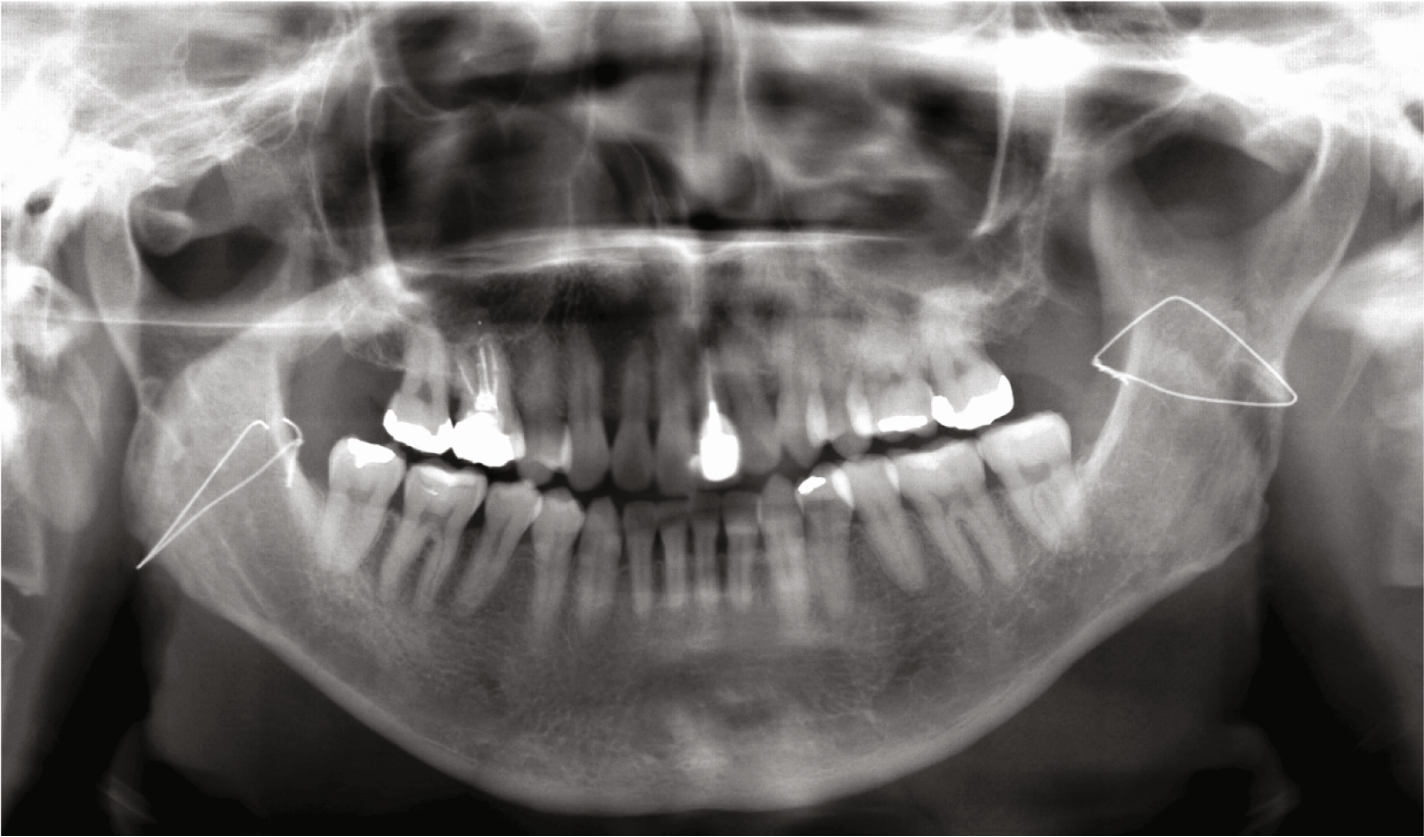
Panoramic radiograph shows normal findings except for the wires of prior orthognathic surgery at the mandibular rami.
